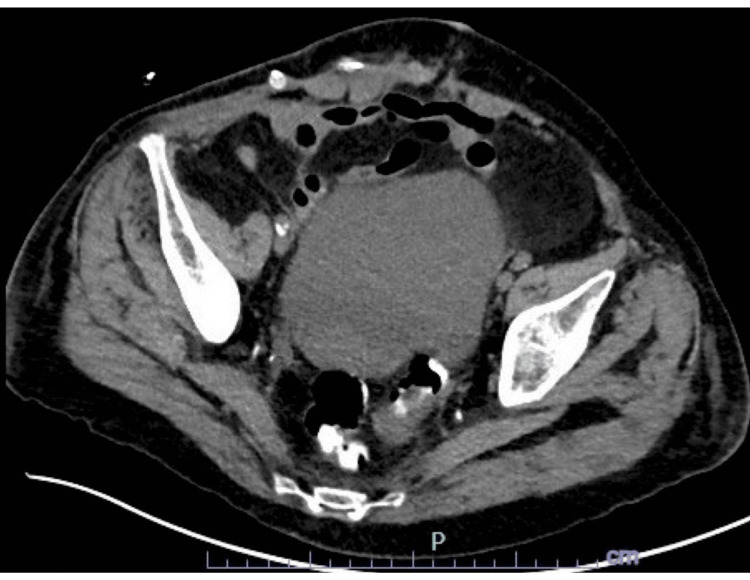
Coronal CT image showing an incarcerated hernia sac contributing to bowel obstruction
